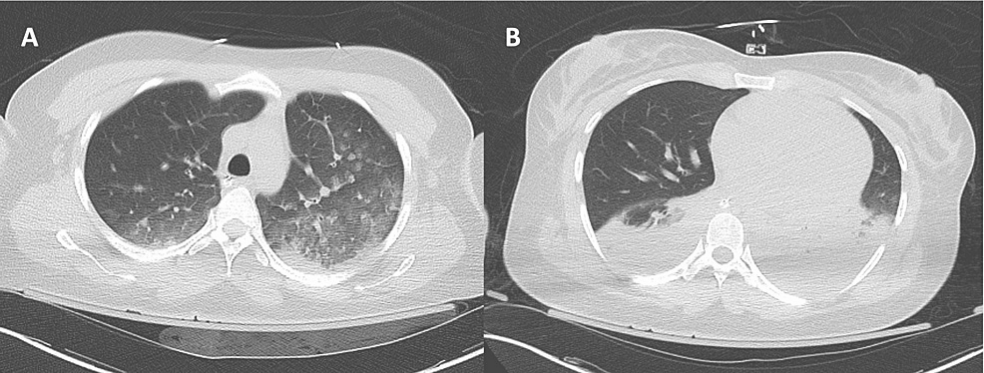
Computed tomography of the chest without contrast A, Ground-glass opacities in the bilateral upper lobes (left greater than right) compatible with pneumonia, including COVID pneumonia. B, Atelectasis/consolidation of the lower lobes, which is also suspicious for pneumonia, including aspiration pneumonia.
